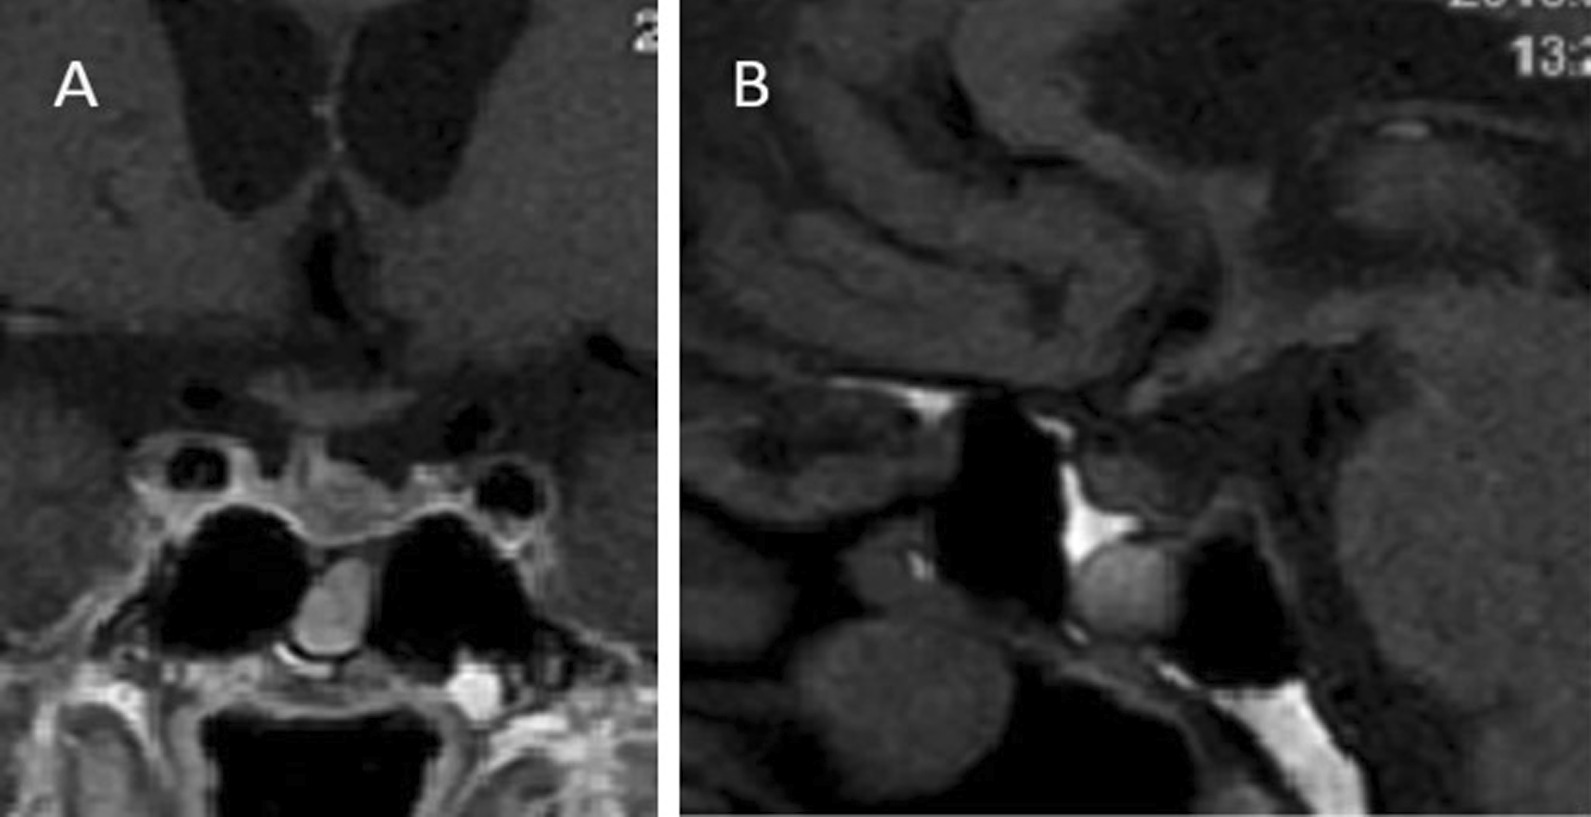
First post-treatment sellar MRI reevaluation in September 2018 demonstrating a significant volume decrease of the lesion, with a paramedian left 8 × 6 × 6 mm remnant, without alterations in the surrounding structures, in coronal (A) and lateral (B) projection
